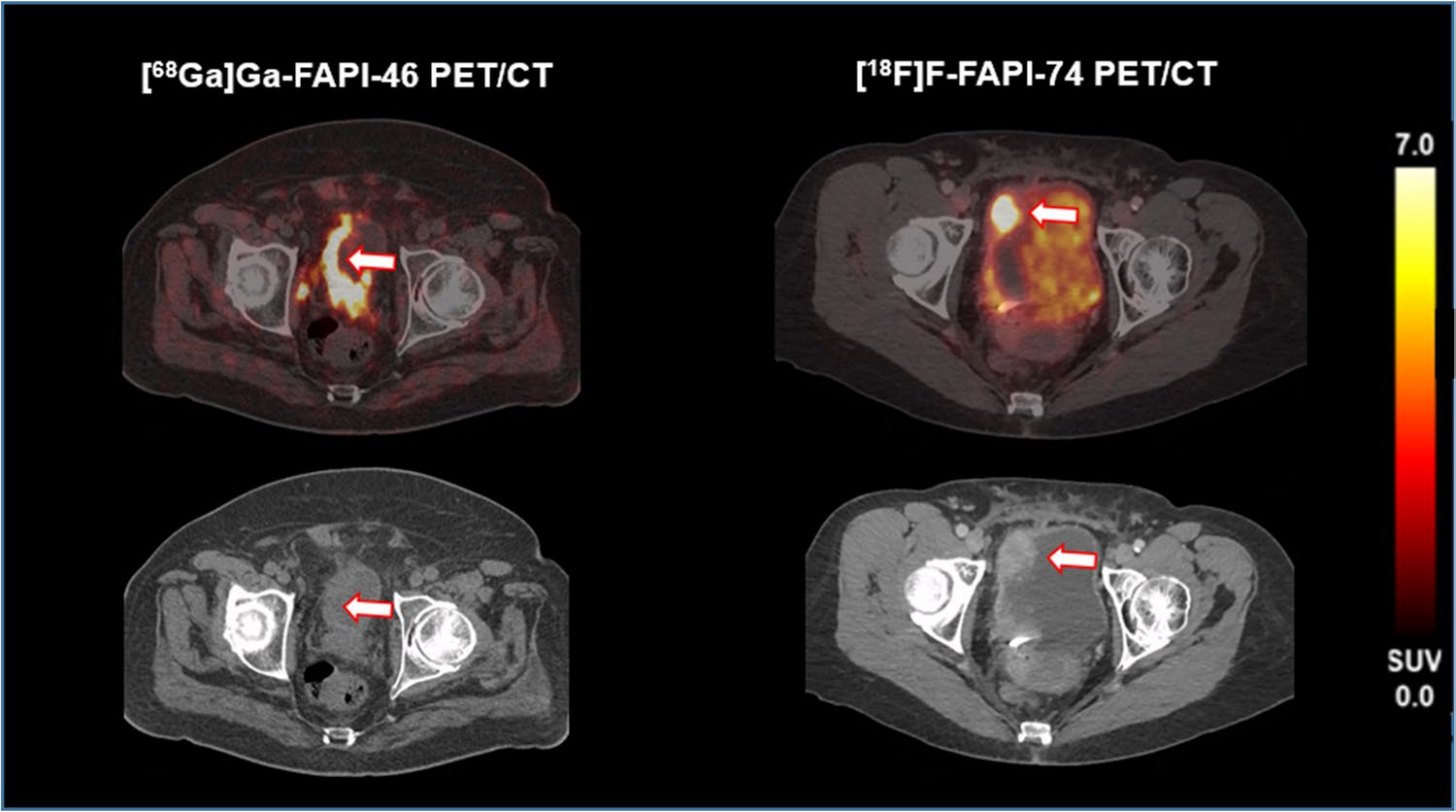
Increased FAPI uptake in the primary tumor in both tracers, Left: [^68^Ga]Ga-FAPI- 46 PET/CT FAP expressing primary (SUV_max_ 21, SUV_mean_ 16); Right: [^18^F]F-FAPI- 74 PET/CT FAP expressing primary (SUV_max_ 24, SUV_mean_ 19). Low dose CT on the left shows an asymmetric thickening of the bladder (arrow), contrast enhanced CT on the right side shows focal tumor tissue (arrow), both findings corresponding with the primary
